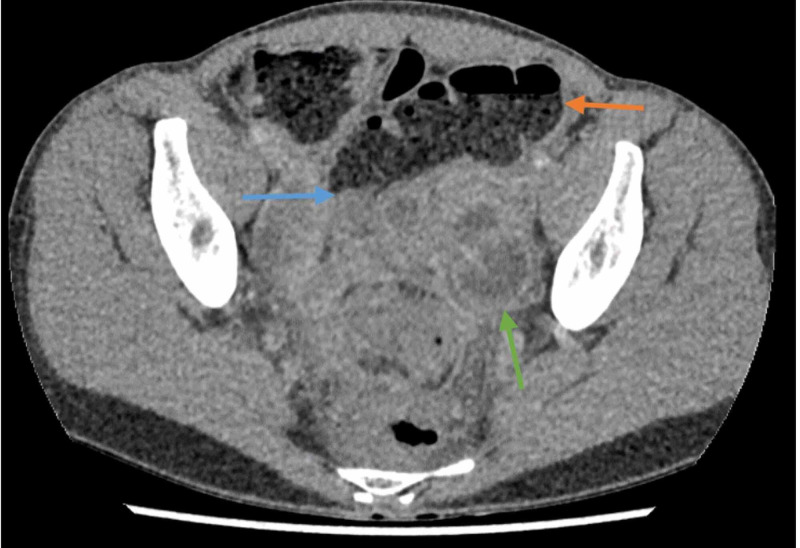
Axial portal venous phase CT of the abdomen and pelvis. Note the transition point (blue arrow) causing proximal dilatation of the sigmoid colon. There are multiple regions of low signal with enhancing rims, representing abscesses (green arrow).
